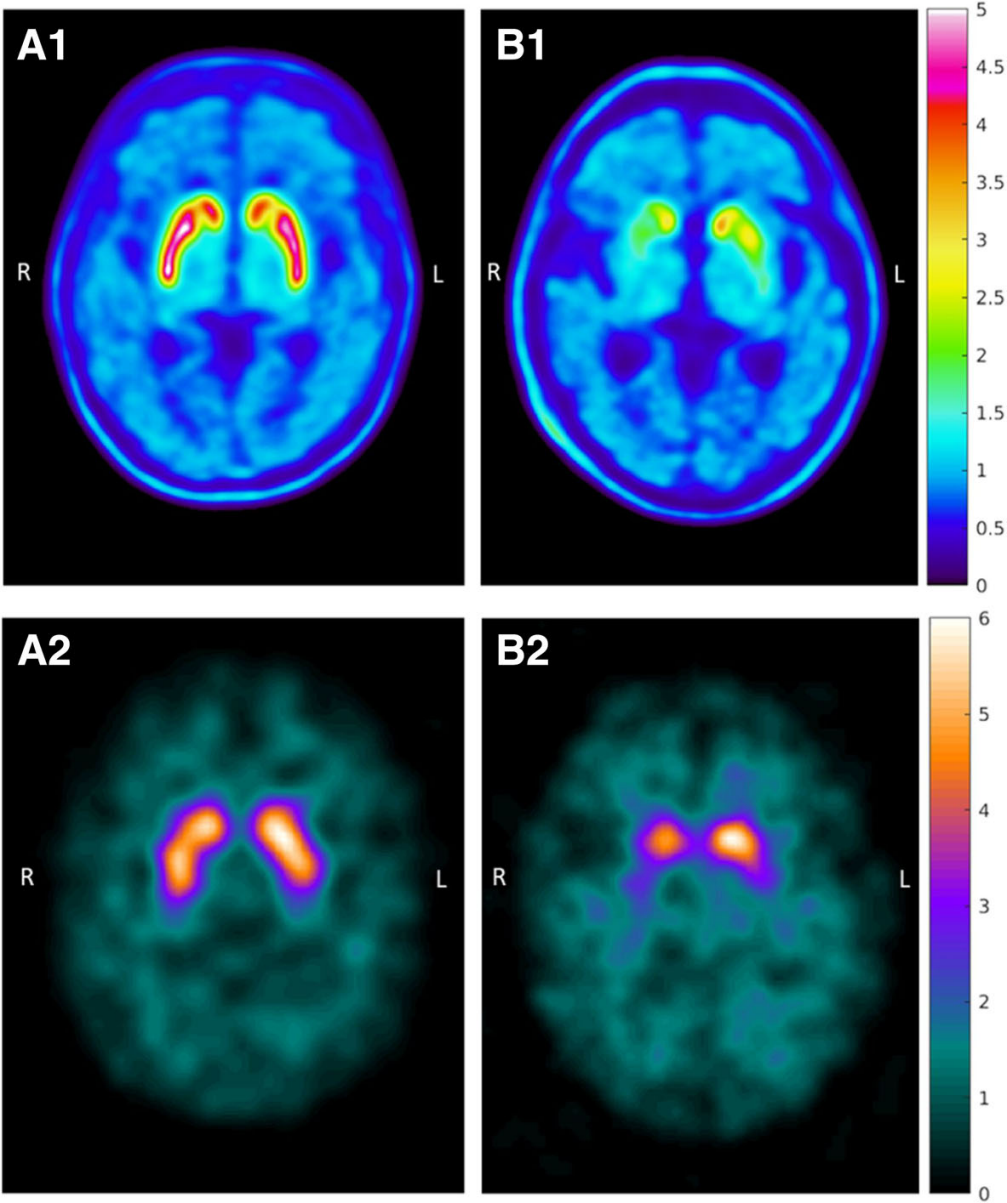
Examples of [^18^F]FE-PE2I PET and [^123^I]FP-CIT SPECT images in the same individuals. Representative images of FE-PE2I PET (parametric DVR images) and FP-CIT SPECT (SUR images) of the same two subjects. Healthy control examined A1 with FE-PE2I and A2 with FP-CIT. Patient with parkinsonian syndrome since 6 months examined B1 with FE-PE2I PET and B2 with FP-CIT SPECT. *PET image scale*: DVR (= BP_ND_ + 1). *SPECT image scale*: activity uptake in striatum divided by cerebellum activity
